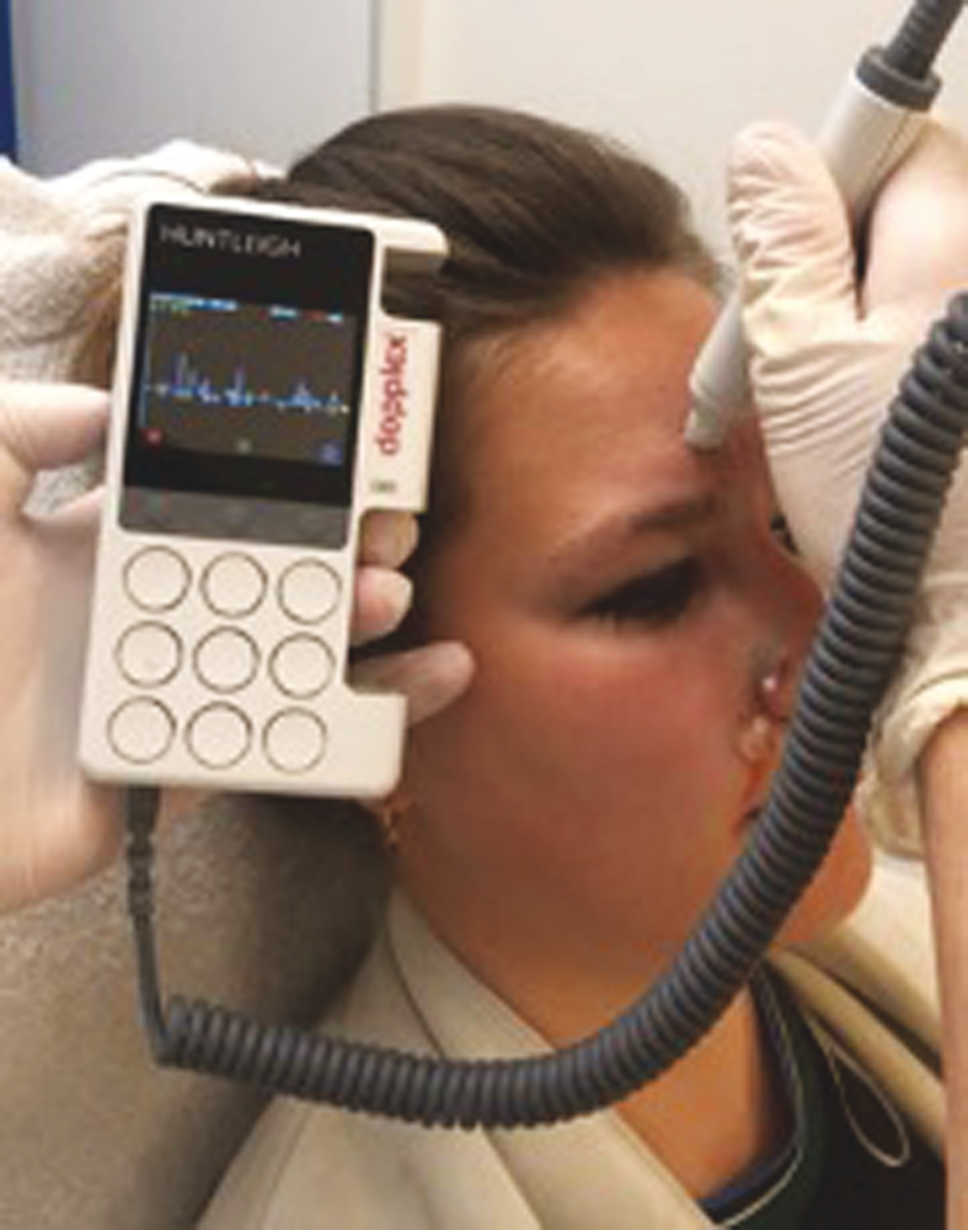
Determining the location of smaller superficial arteries of a 27-year-old female’s face by Doppler, which are not visualized by duplex ultrasound.
